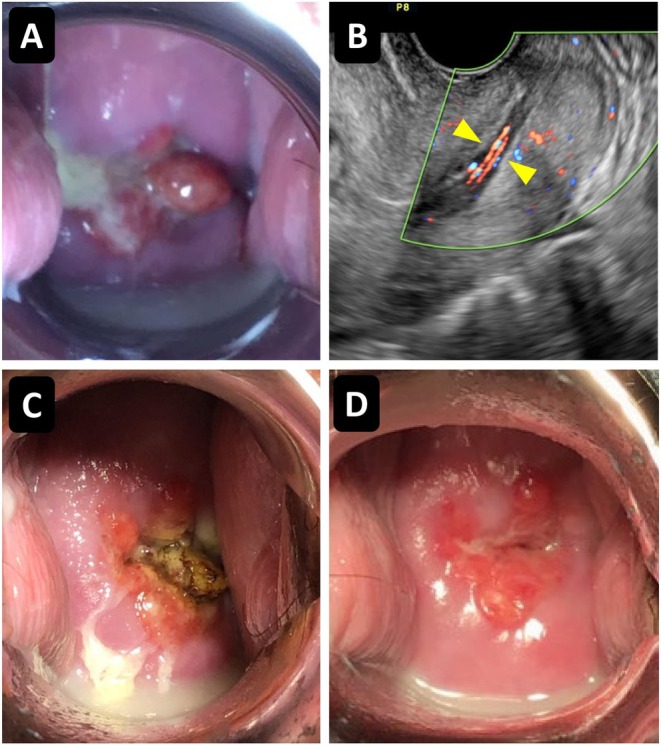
Case 1. (A) At 10 weeks and 4 days of gestation, a cervical polyp protruding from the external cervical os, measuring 10 mm in diameter, was observed. (B) Color Doppler ultrasonography showed blood flow within the cervical canal continuous with the stalk of the cervical polyp (yellow arrows), supporting the diagnosis of a decidual cervical polyp. (C) At 11 weeks and 3 days of gestation, cauterization was performed, consisting of five applications of 2–3 s each, resulting in hemostasis. After cauterization, a superficial eschar formed on the polyp surface, without thermal extension into the cervical canal. (D) At 19 weeks and 5 days of gestation, the cervical polyp was no longer visible on macroscopic examination.
